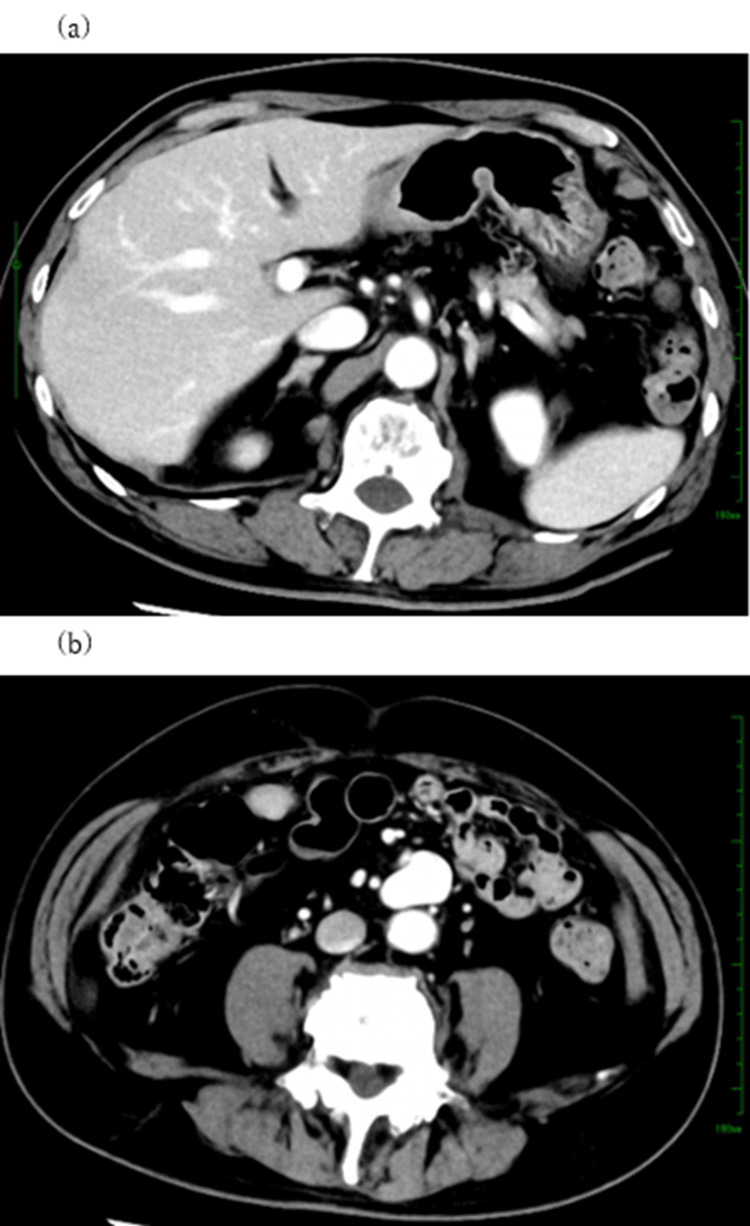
Axial view of the enhanced abdominal CT after one year and nine months. Both an embolus of the intrahepatic portal vein (a) and intraluminal thrombus in the superior mesenteric vein (SMV) aneurysm (b) have disappeared.
